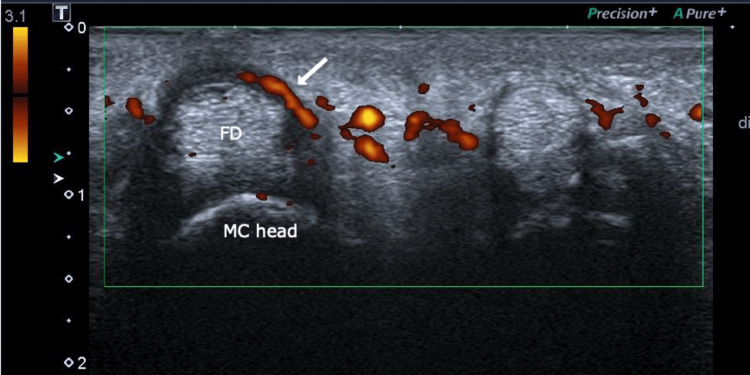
Transverse-view ultrasound scan of volar aspect of the second metacarpophalengeal joint of the right hand showing active tenosynovial power doppler signal (white arrow) FD: Flexor digitorum; MC: Metacarpal
